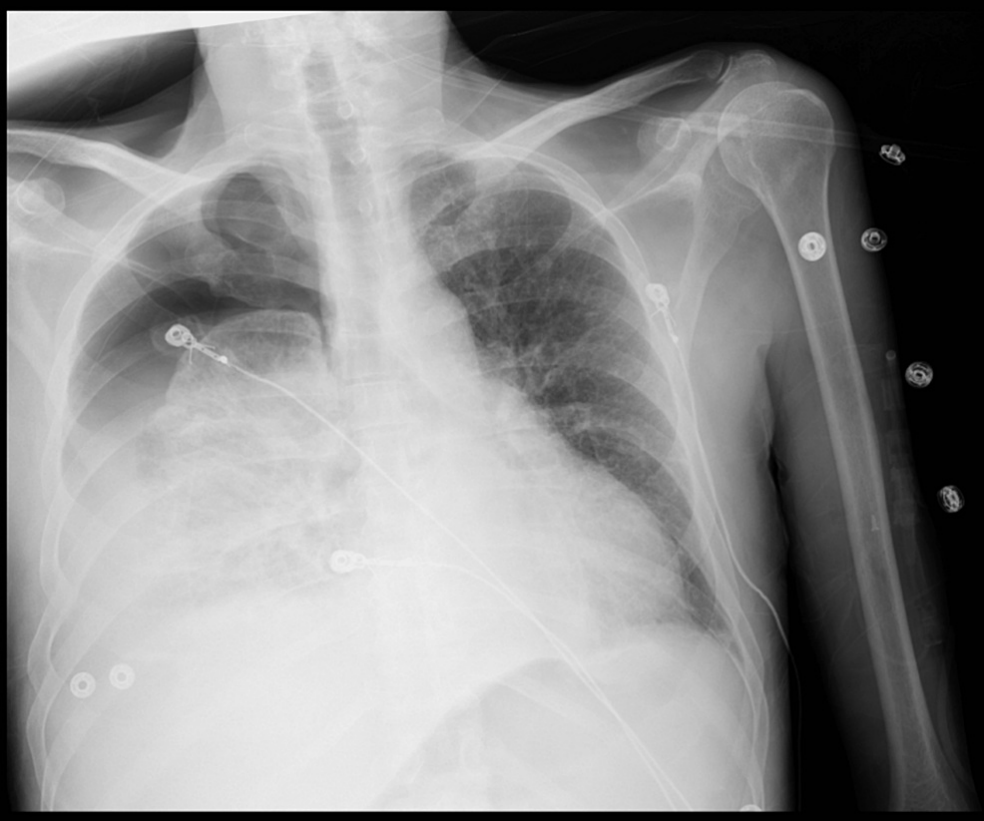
Initial chest X-ray showing right-sided hydropneumothorax
